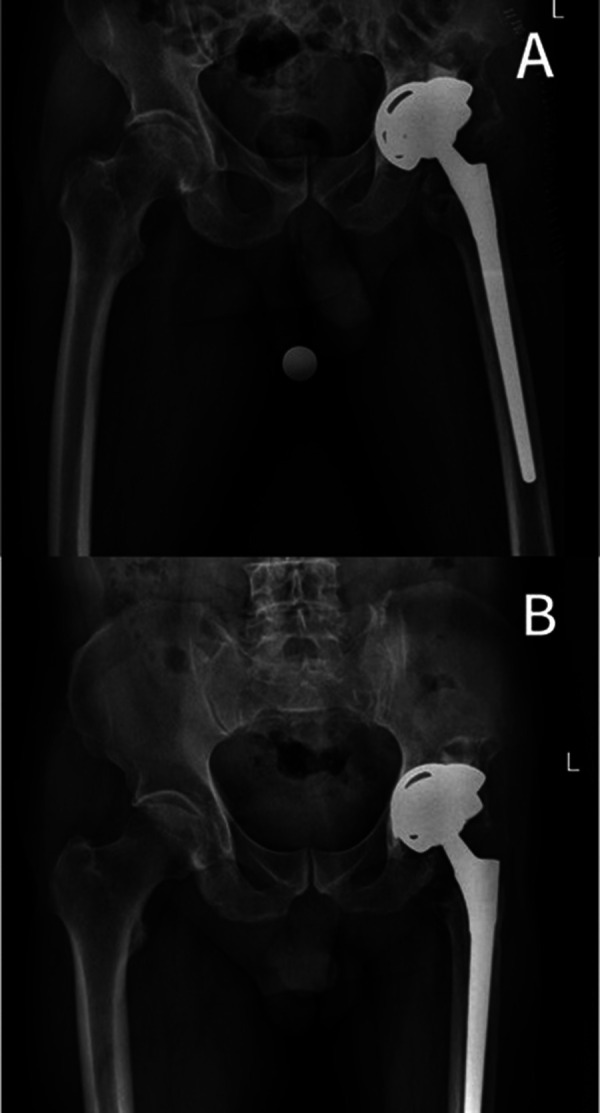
(A) Postoperative radiographs. (B) Radiographs at the last follow-up.
